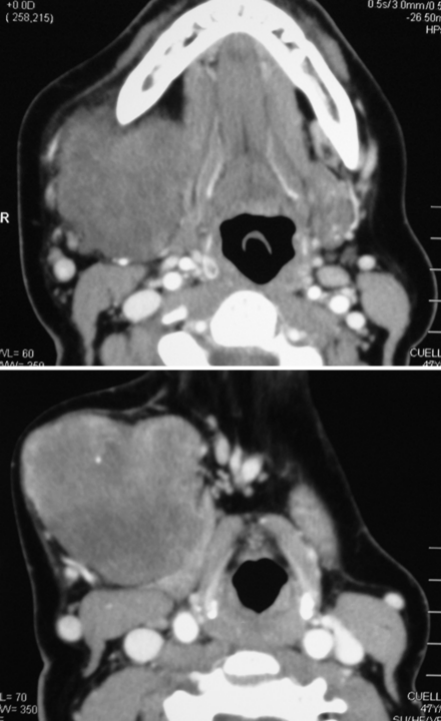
Preoperative computed tomography scan demonstrating a large well-defined mass without cystic changes or necrotic areas in the right submandibular region.
